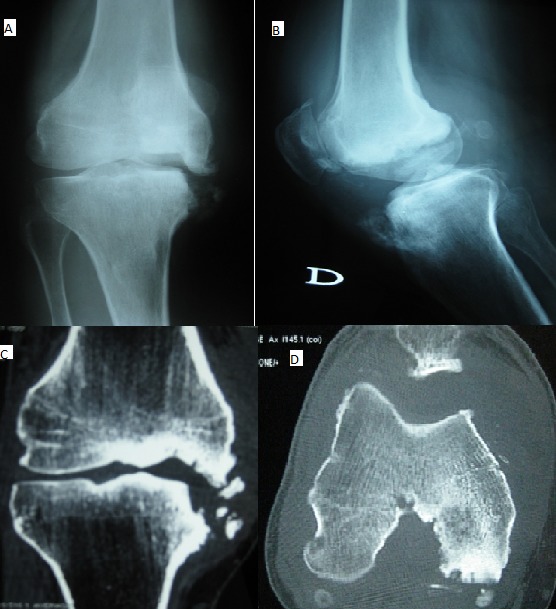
(A,B) radiographie du genou droit de face et de profil: destruction du condyle fémoral interne avec ostéolyse du plateau tibial interne associé à de multiples constructions osseuses; (C,D) scanner du genou droit en coupe sagittale et axiale: destruction du condyle fémoral interne avec ostéolyse du rebord médial du plateau tibial interne associé à de multiples constructions osseuses avec présence de fragments intra-articulaires, d’un épanchement intra-articulaire et d’un épaississement de la synoviale
